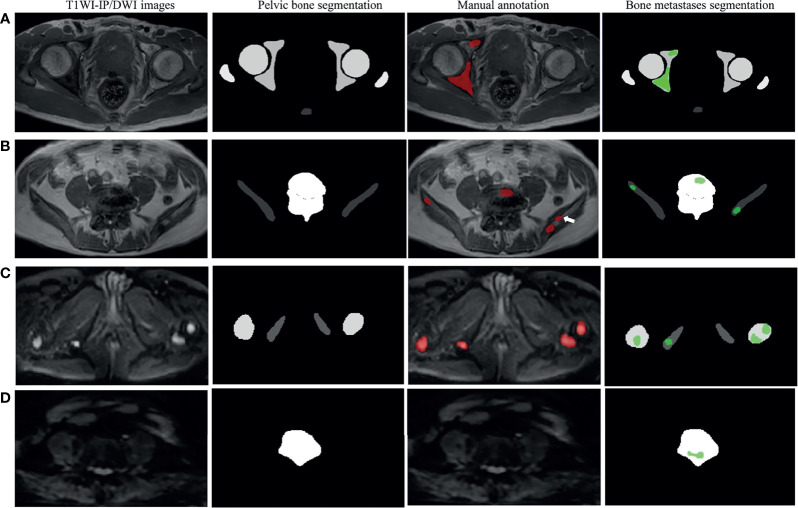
Examples of pelvic bony structure and bone metastases segmentations. (A) Two metastases of acetabulum annotated by radiologists were corrected segmented by the model on T1WI-IP images (true positive). (B) Four of five metastases annotated by the radiologists were corrected segmented by model on T1WI-IP images; one metastasis on the right ilium was missed (the white arrow pointed, false negative). (C) All the four metastases of femoral head and ischium annotated by radiologists were correctly segmented by the model on DWI images (true positive). (D) One metastasis of lumbar vertebra was segmented by the model by error, which was not annotated by the radiologists (false positive). T1WI-IP, T1W images obtained using the Dixon technique with in-phase.
